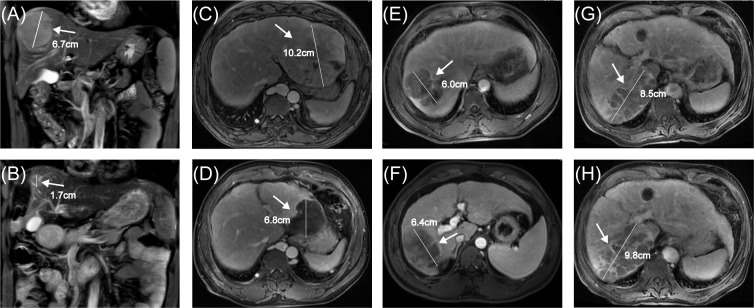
Tumor responses on CE-MRI in patients with advanced HCC and MVI treated with Icaritin plus TACE. Patient 1 (A, B): A 53-year-old male with a 20-year HBV history. Baseline CE-MRI revealed a lesion in the superior right hepatic lobe (6.7 cm; A). After six cycles of Icaritin + TACE, the lesion regressed to 1.7 cm with no viable tumor at month 16. Response: complete response (B). Patient 2 (C, D): A 52-year-old male with HCC. Initial MRI showed multiple lesions in the left lobe, the largest measuring 10.2 cm with intense arterial enhancement (C). After five cycles, MRI at month 15 showed shrinkage to 6.8 cm with partial enhancement. Response: partial response (D). Patient 3 (E, F): A 47-year-old male with >10-year HBV history. Baseline MRI showed a 6.0 cm lesion in the right posterior lobe and tumor thrombus in the IVC and hepatic vein (E). After five cycles, enhancement and thrombus persisted. Response: stable disease (F). Patient 4 (G, H): A 60-year-old male with a 40-year HBV history. Pre-TACE MRI showed multiple hepatic lesions, including an 8.5 cm dominant mass (G). After five cycles, the lesion enlarged to 9.8 cm. Response: progressive disease (H). CE-MRI, contrast-enhanced magnetic resonance imaging; HCC, hepatocellular carcinoma; MVI, macrovascular invasion; TACE, transarterial chemoembolization; HBV, hepatitis B virus.
